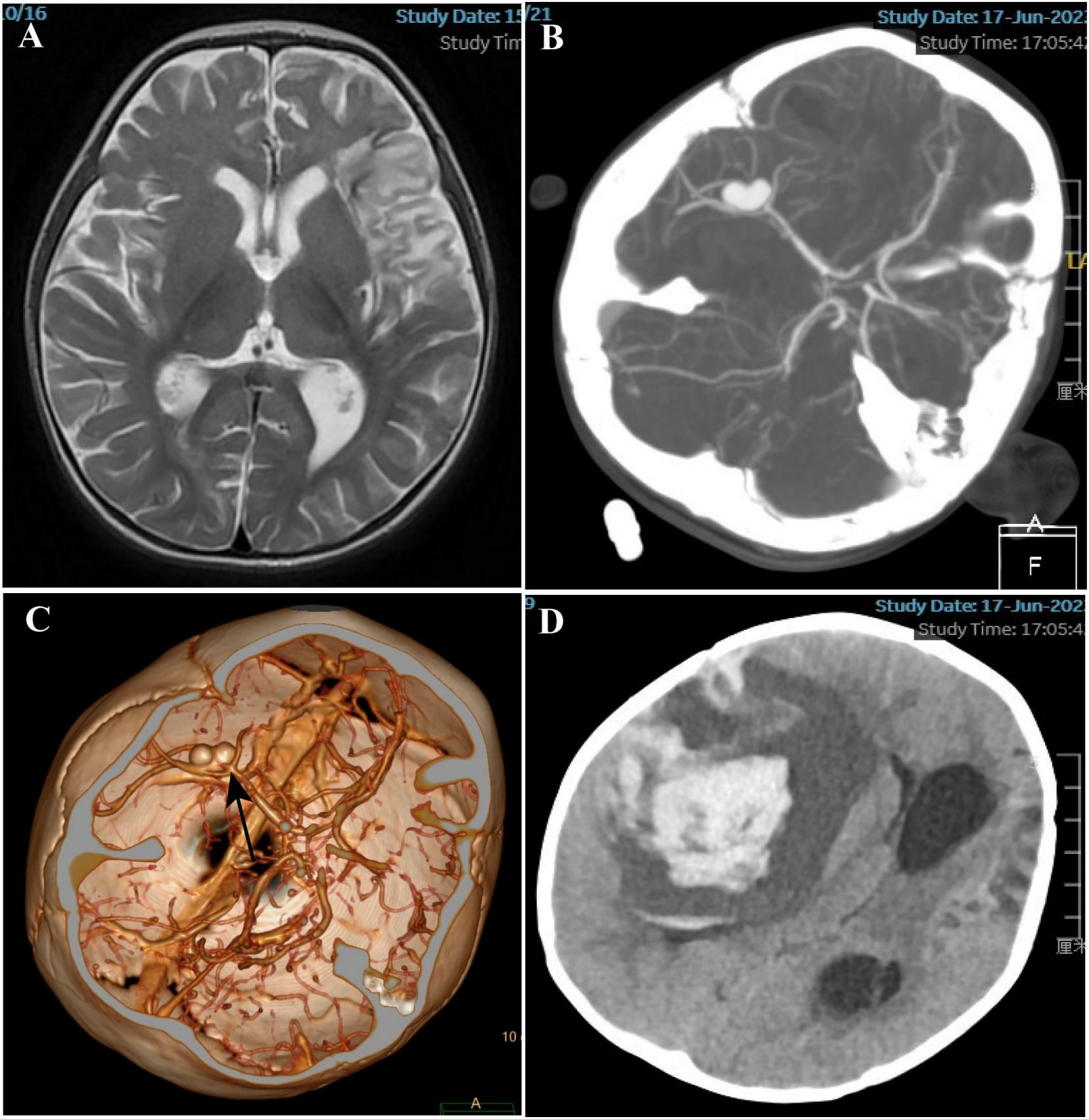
(A) MRI image 6days after embolization. (B, C) CTA scan immediately following the seizure attack 7days after embolization and the newly formed Infectious intracranial aneurysms (IIA) presented (black arrow). CT scan presented a massive hematoma located in the right basal ganglia. CTA, computed tomography angiography; MRI, magnetic resonance imaging.
